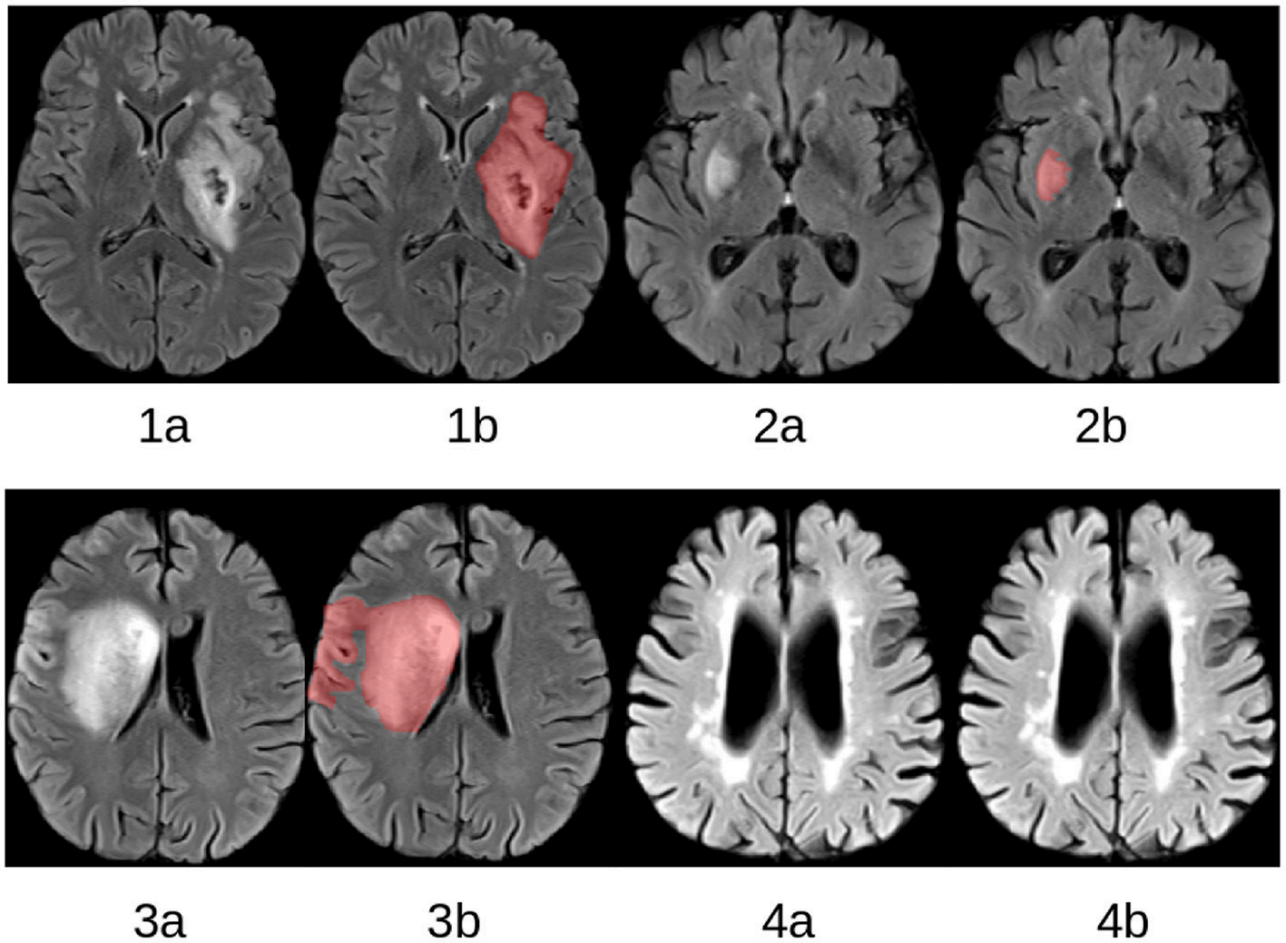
Selected slices from four FLAIR MRI datasets (1a–4a) with corresponding expert lesion segmentations (1b–4b).The lesions vary considerably with respect to shape, position, and size. Patient 4 does not display a lesion resulting from an acute ischemic stroke but considerable white matter hyperintensities, which are often falsely segmented by automatic lesion segmentation methods.
